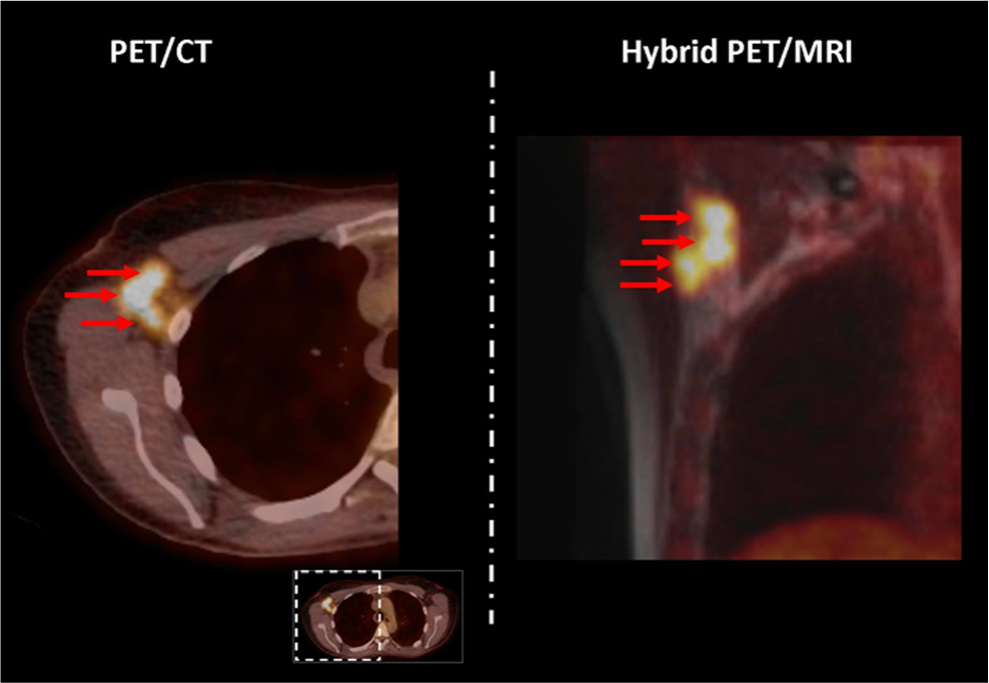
Example of a patient with a 35-mm invasive carcinoma of no special type in her right breast. One cross-sectional PET/CT image (*left*) of the right axilla demonstrates three suspicious lymph nodes (*red arrows*) out of a total of five suspicious lymph nodes on PET/CT. One coronal dedicated axillary hybrid PET/MRI image (*right*) demonstrates four suspicious lymph nodes (*red arrows*), with a total of eight suspicious lymph nodes on dedicated axillary hybrid PET/MRI. The most dorsally located suspicious lymph node on (low-dose) PET/CT consists of two suspicious lymph nodes on dedicated axillary hybrid PET/MRI
